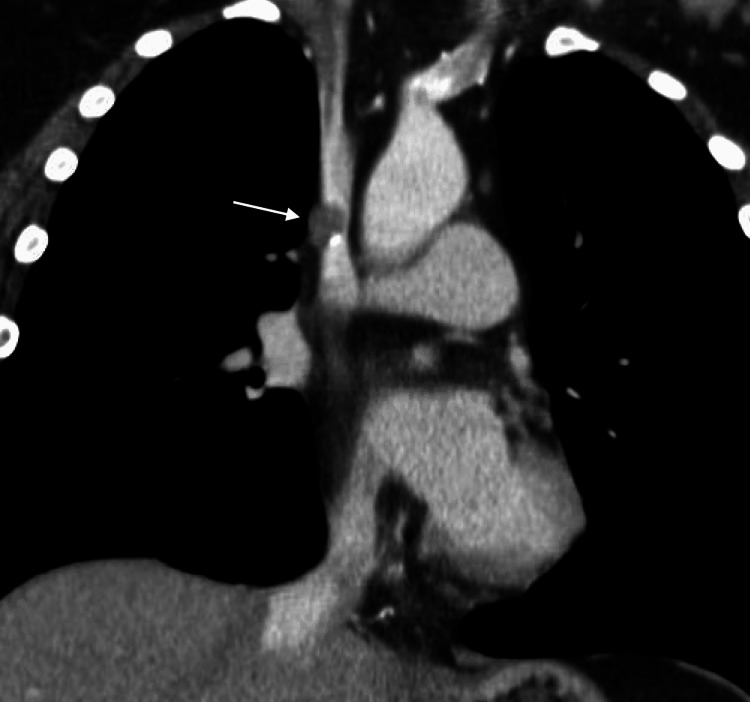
Coronal view of a CT chest showing another view of the eccentric, partially calcified, hypodense nodule (1.1 x 1.4 cm, white arrow) abutting and encroaching into the lower SVC CT: Computed Tomography, SVC: Superior Vena Cava
